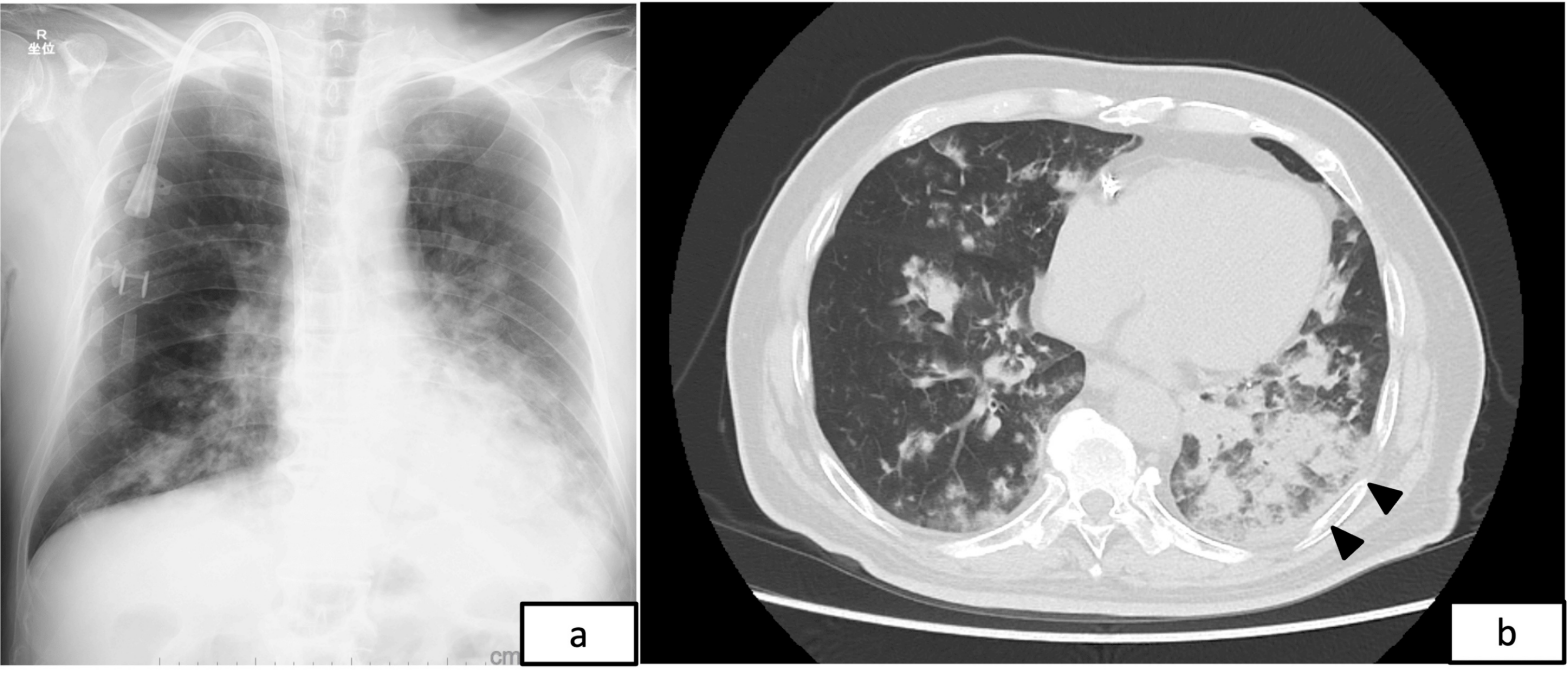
Chest radiograph (a) and computed tomography (b) on admission revealing bilateral infiltrative opacities, predominantly in the lower lung fields and bases. The hemodialysis catheter is visible on chest radiography.
